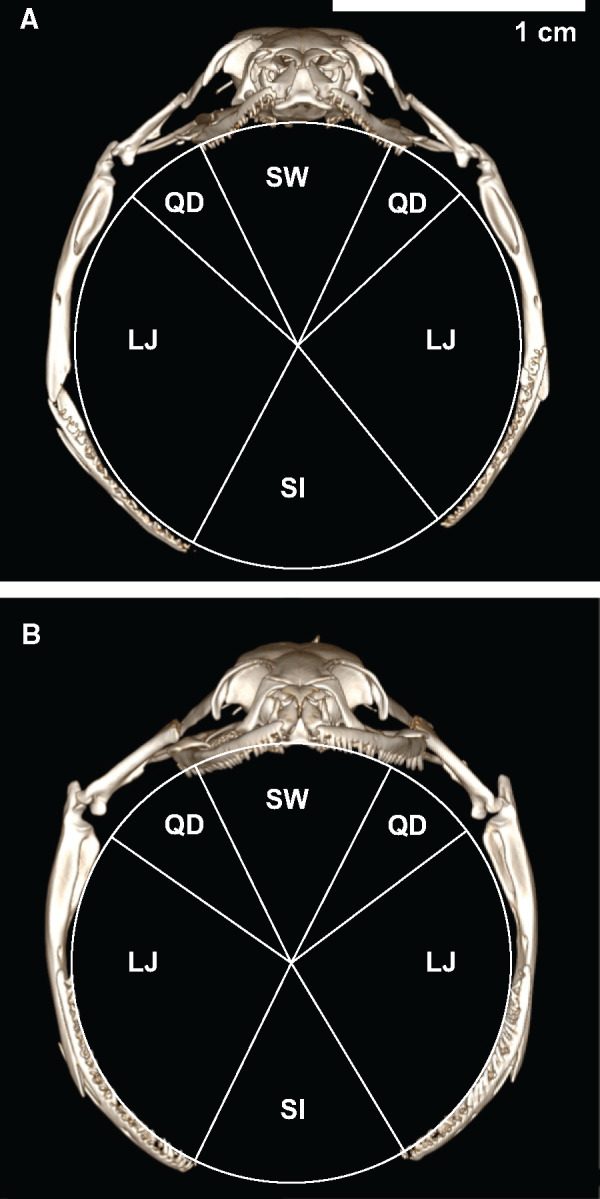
Contributions of skeletal elements and soft tissues to maximal gape area. Anterior views of computed tomography scans of *R. septemvittata* (A) and *L. alleni* (B) preserved at maximum gape. The relative contributions to maximal gape area are shown for the skull width (SW), quadrate (QD), lower jaw (LJ), and the skin and other intermandibular soft tissues between the lower jaws (SI). See Table 2 for mean values and comparisons between species.
